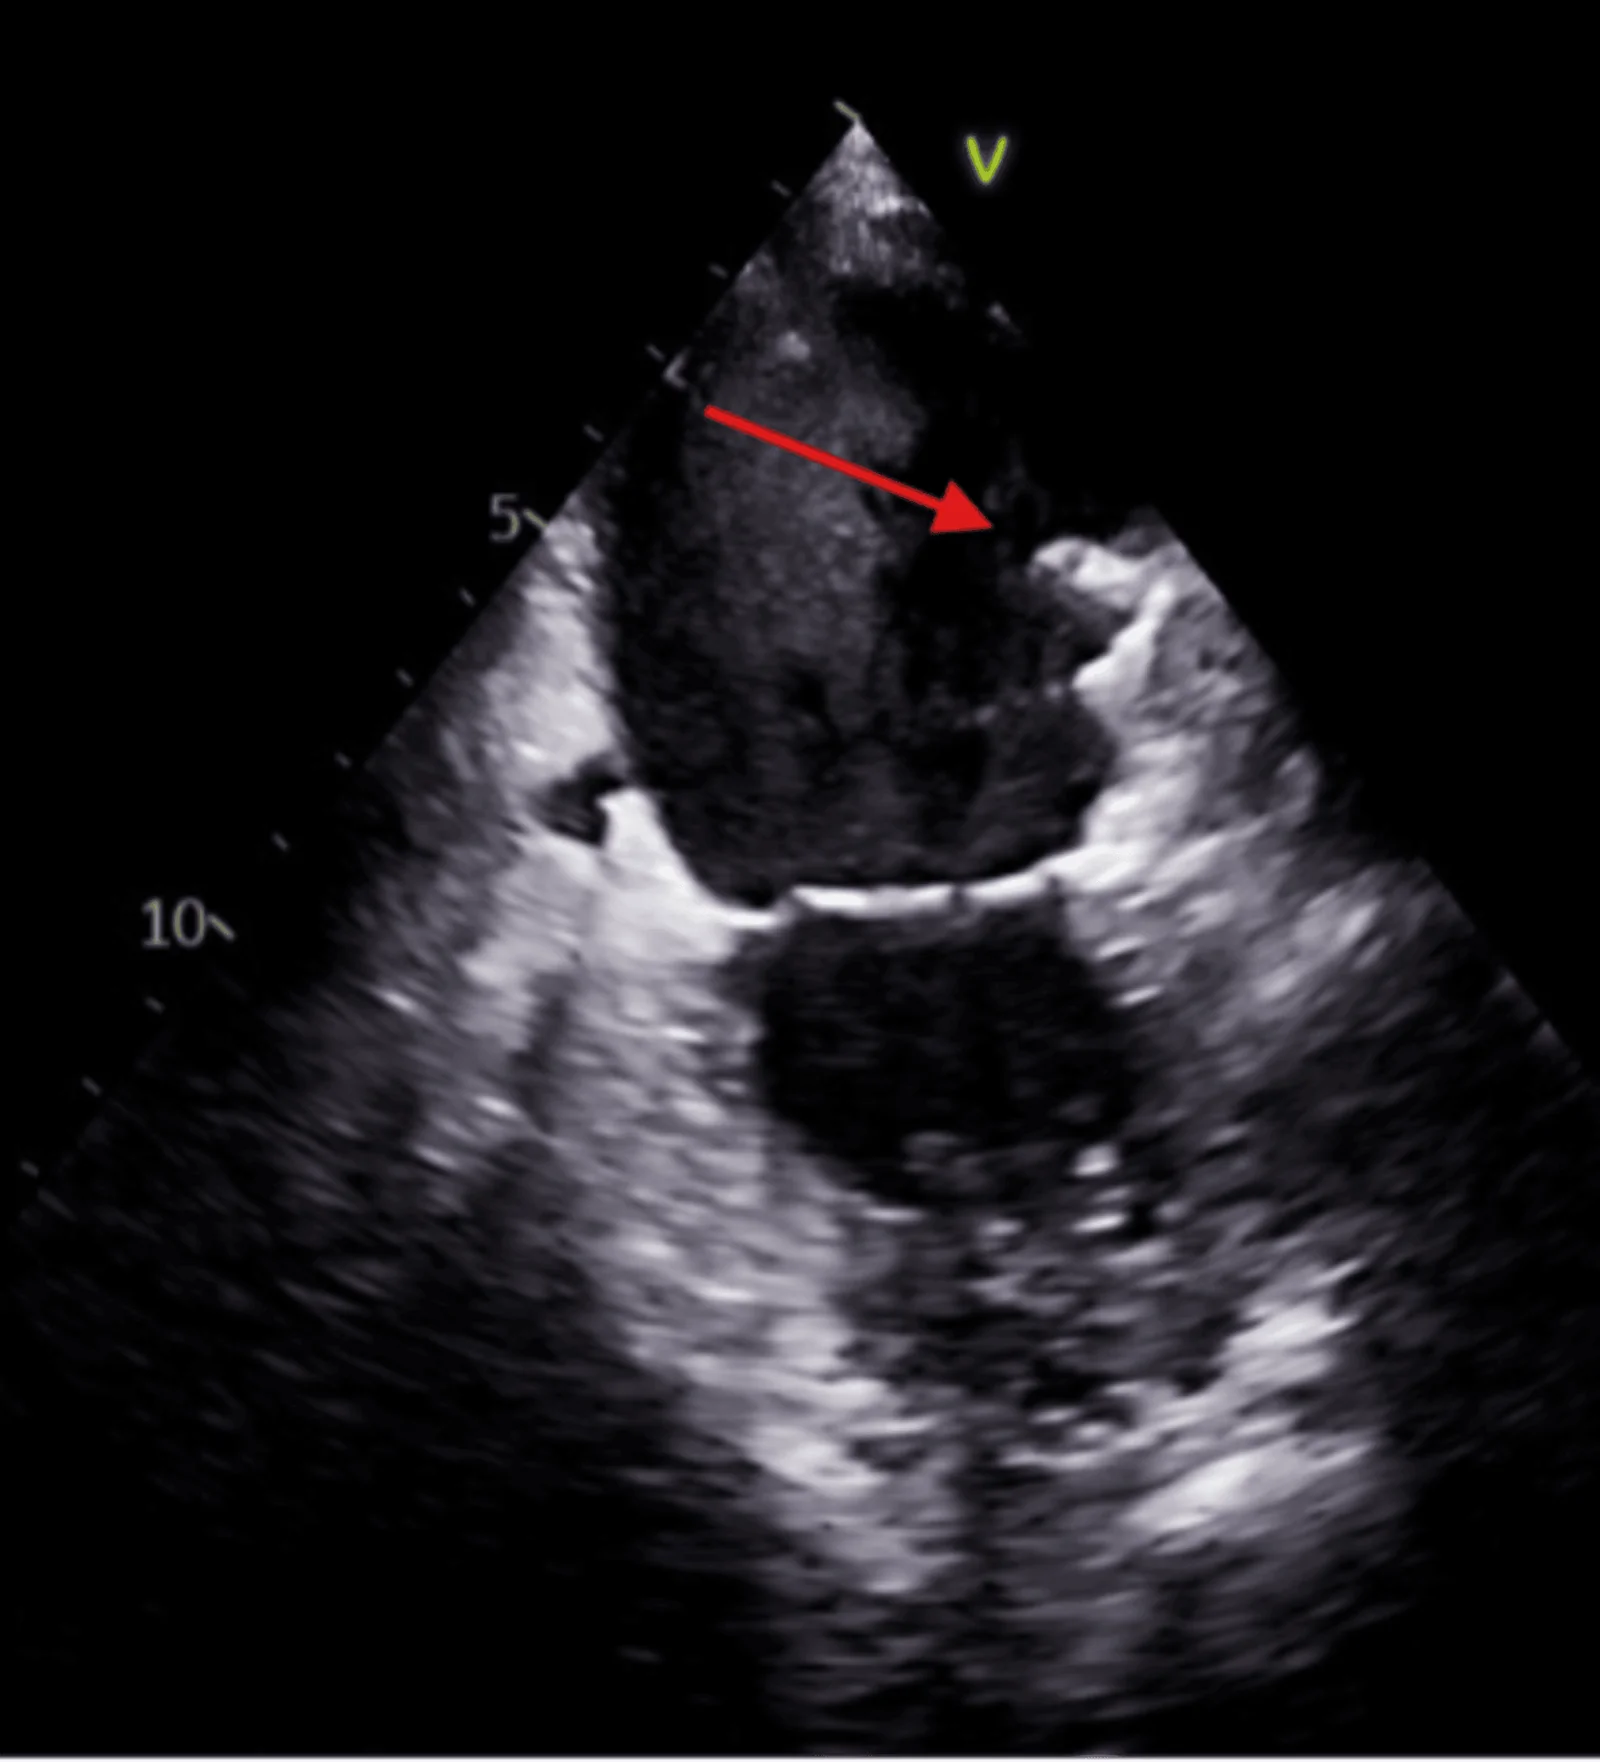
LAAO device. TEE image showing the patient’s LAAO device (arrow). LAAO, left atrial appendage occlusion; TEE, transesophageal echocardiography
